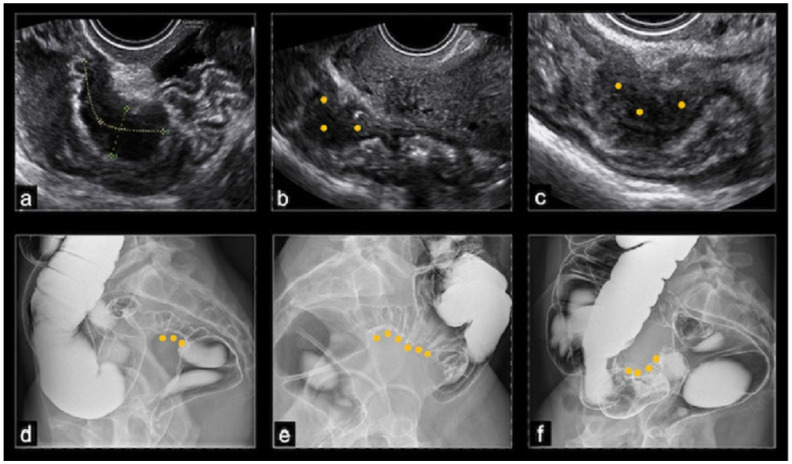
(a–c) Ultrasonographic images of rectal nodules causing an estimated stenosis of 30–40%; (d) barium enema image of a recto-sigmoid junction nodule causing an estimated stenosis of 30–50%; (e) barium enema image of a sigmoid nodule causing an estimated stenosis greater than 50%; (f) barium enema image showing a cecal nodule.
